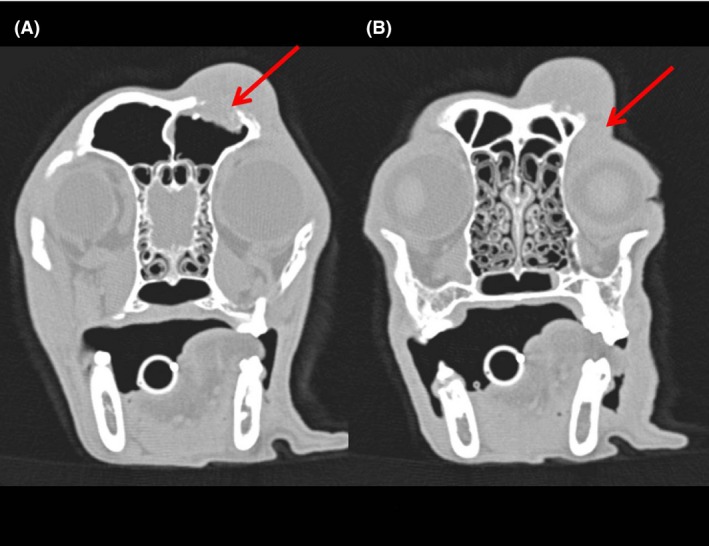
Computed tomography. Transversal plane. Focally extensive lysis and erosion of the bone. (A) Extension of the mass into the frontal sinus (red arrow). (B) Mild extension of the mass into the orbit (red arrow).
